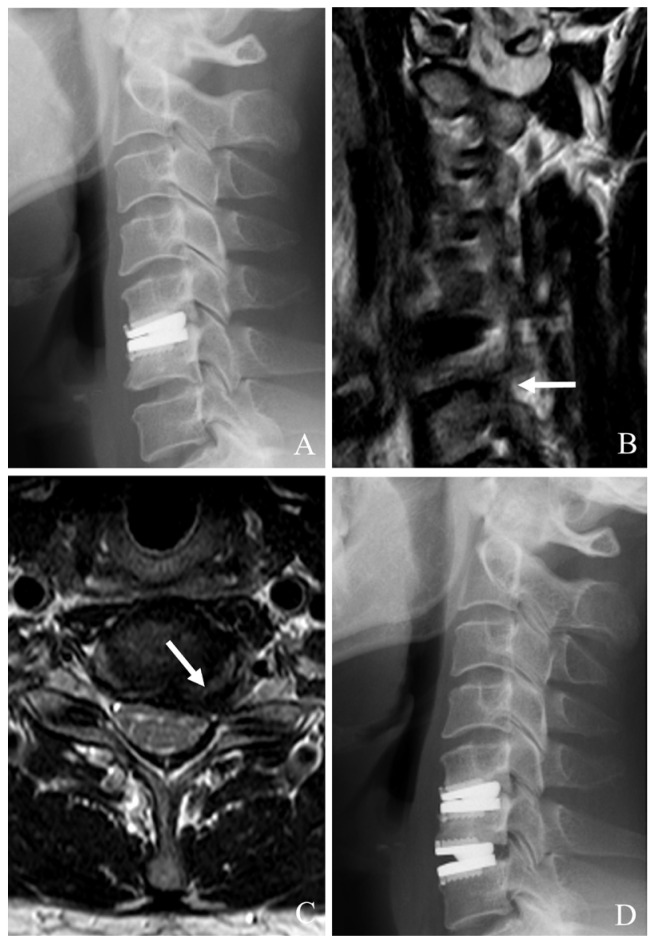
Plain X-rays (A), magnetic resonance image (B,C) showing kyphosis of C5–6 prosthesis and C6–7 adjacent segment disease with left foraminal stenosis and disc herniation (white arrows). The patient underwent removal of C6–7 redo cervical disc arthroplasty (D).
